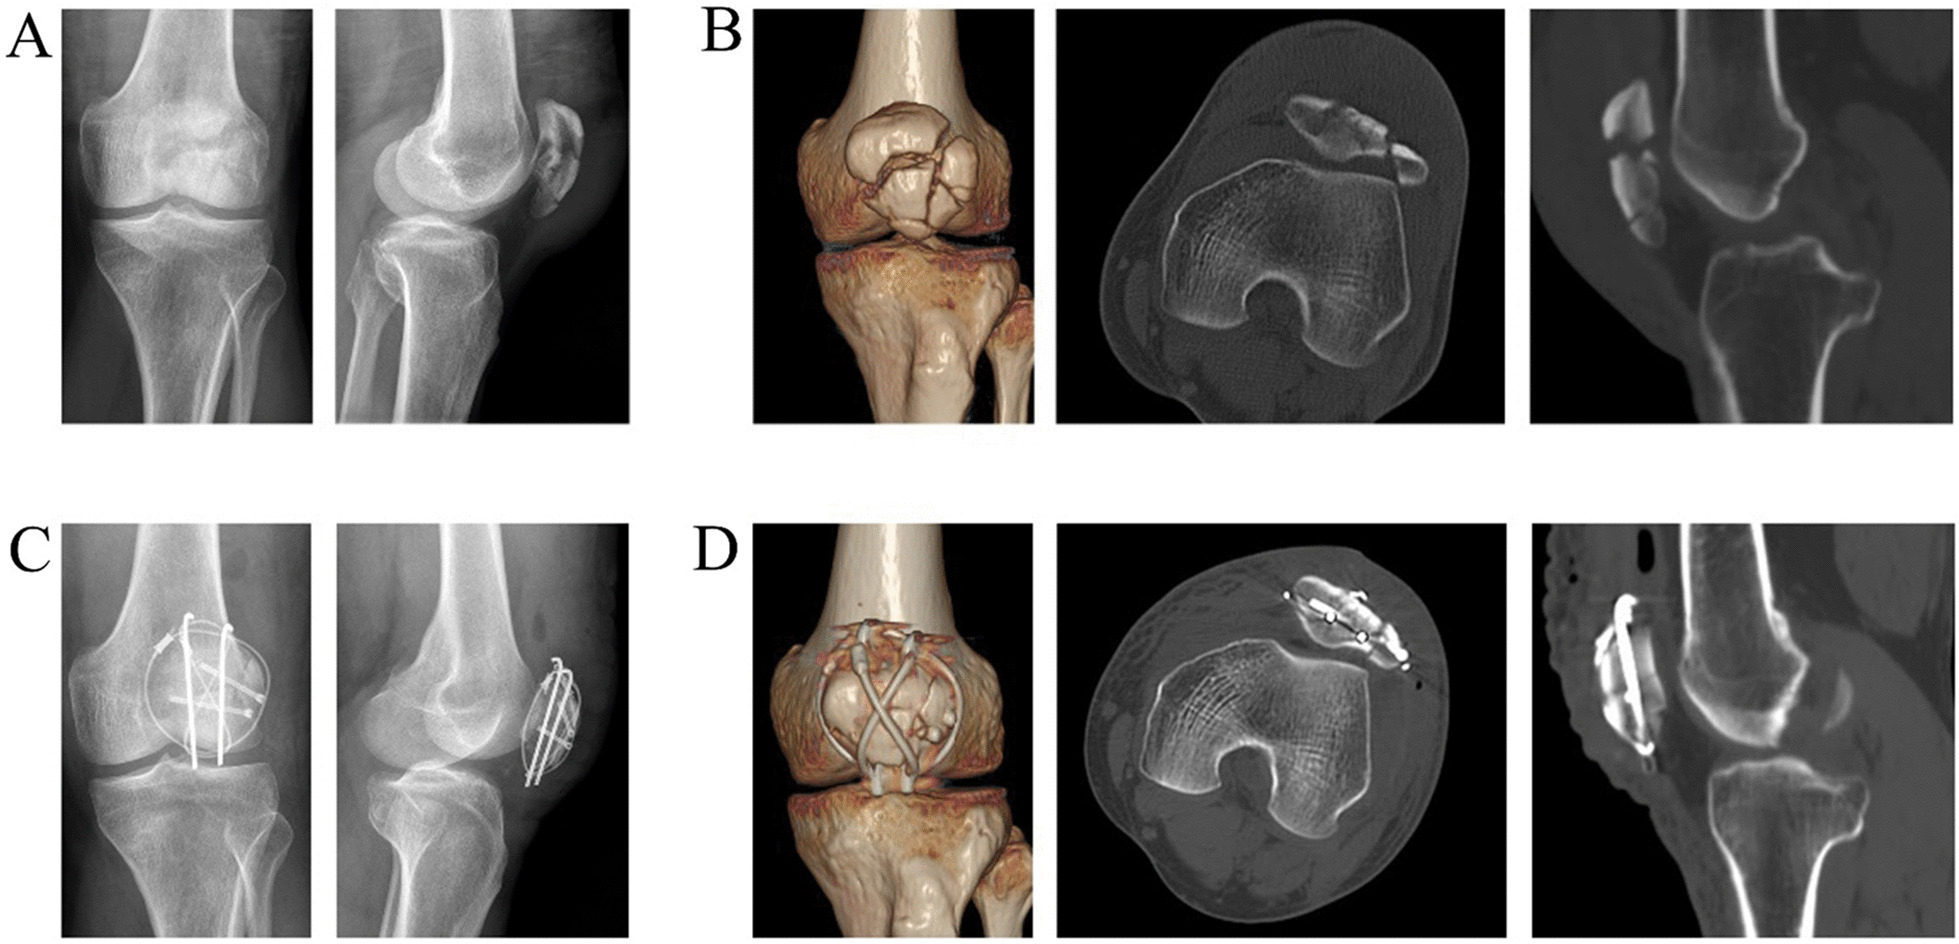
Pre- and post-operative imaging of a 57-year-old male are shown. The patient's preoperative x-ray and CT results were shown in A, B, while the post-operative x-ray and CT results were in C, D
